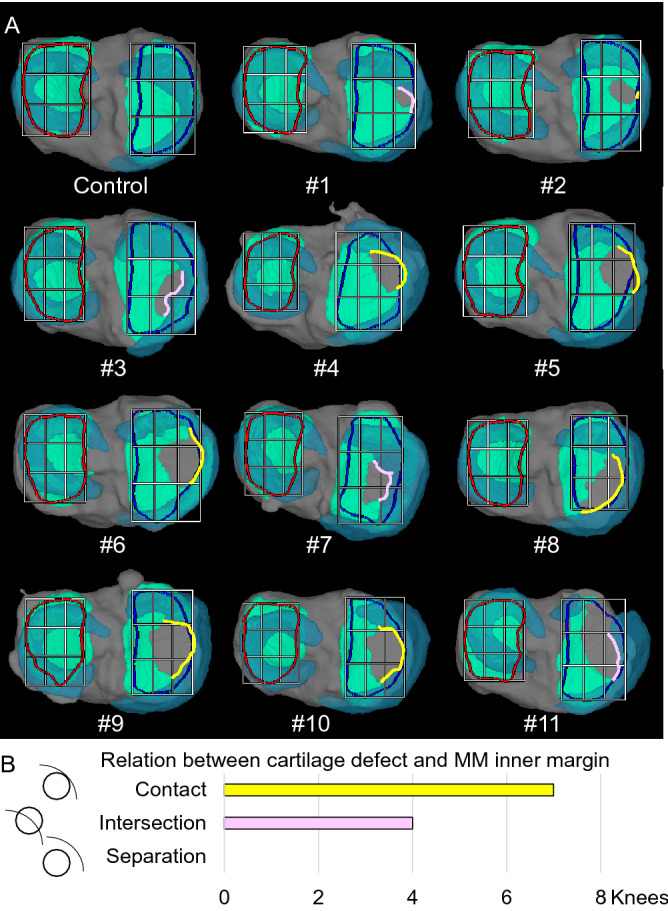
Relationship between cartilage defects and the inner margin of the medial meniscus (MM). (A) 3D MRI images of the articular surface of the tibia. The tibial cartilage is shown in green, the meniscus in translucent blue, and the bone in gray. The line where the inner margin of the MM contacts the cartilage defect is shown in yellow, and the line where the inner margin of the MM crosses the cartilage defect is shown in pink. (B) Quantitative evaluation of the relationship between cartilage defects and the inner margin of the MM.
